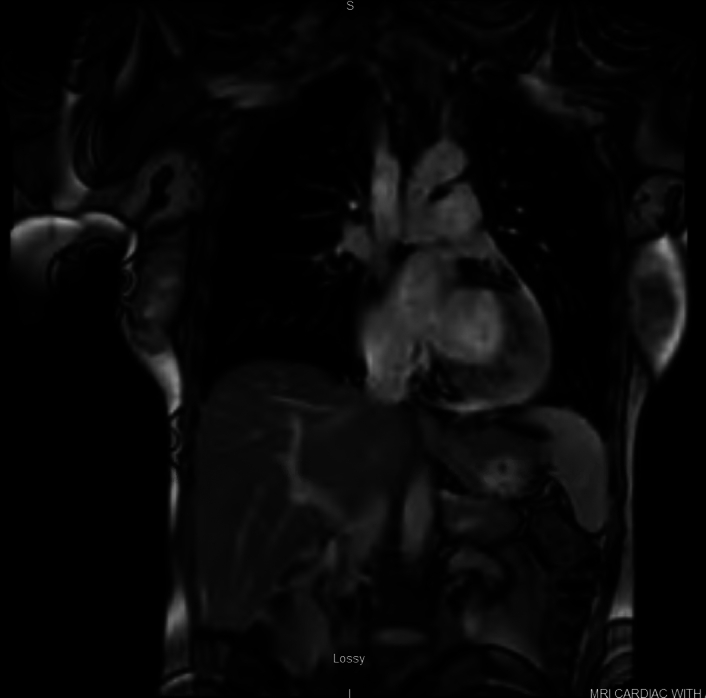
Cardiac MRI, Cardiac MRI demonstrating no evidence of myocardial scarring/fibrosis with no late gadolinium enhancement.
